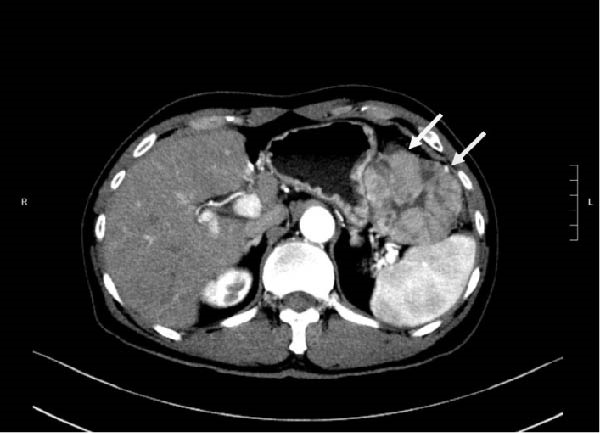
CT showing a peritoneal recurrent tumor near the splenic hilum (arrows).
